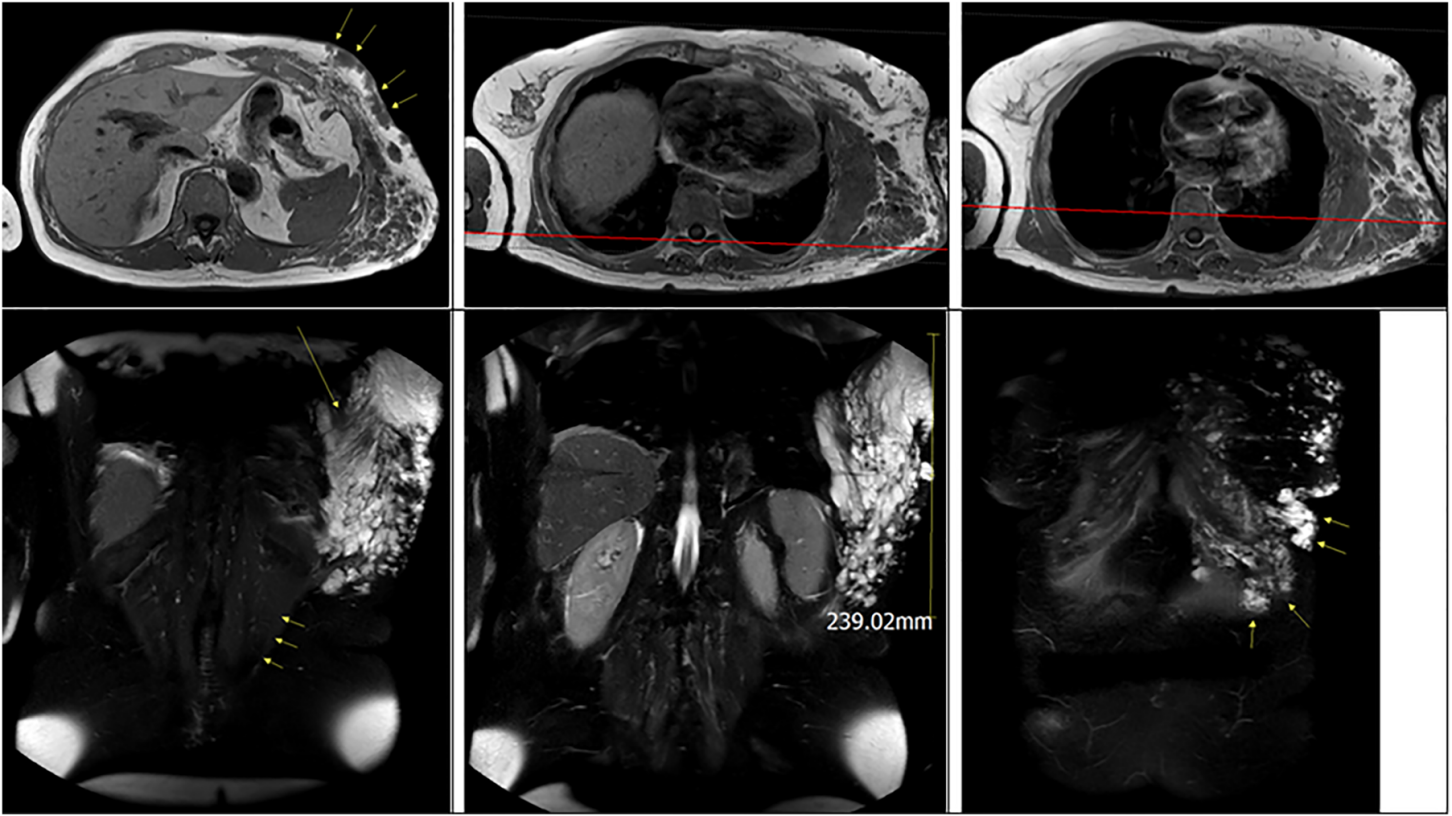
MRI: (A) T1 axial scan – cutaneous site at the arcus; (B) T1 axial scan – finding at the M. latissimus dorsi; (C) T1 axial scan – main finding at the posterior axillar line; (D) T2 coronary scan – M.-latissimus-dorsi line; (E) T2 coronary scan – main finding at the posterior axillar line; (F) T2 coronary scan – cutaneous site at the arcus.
